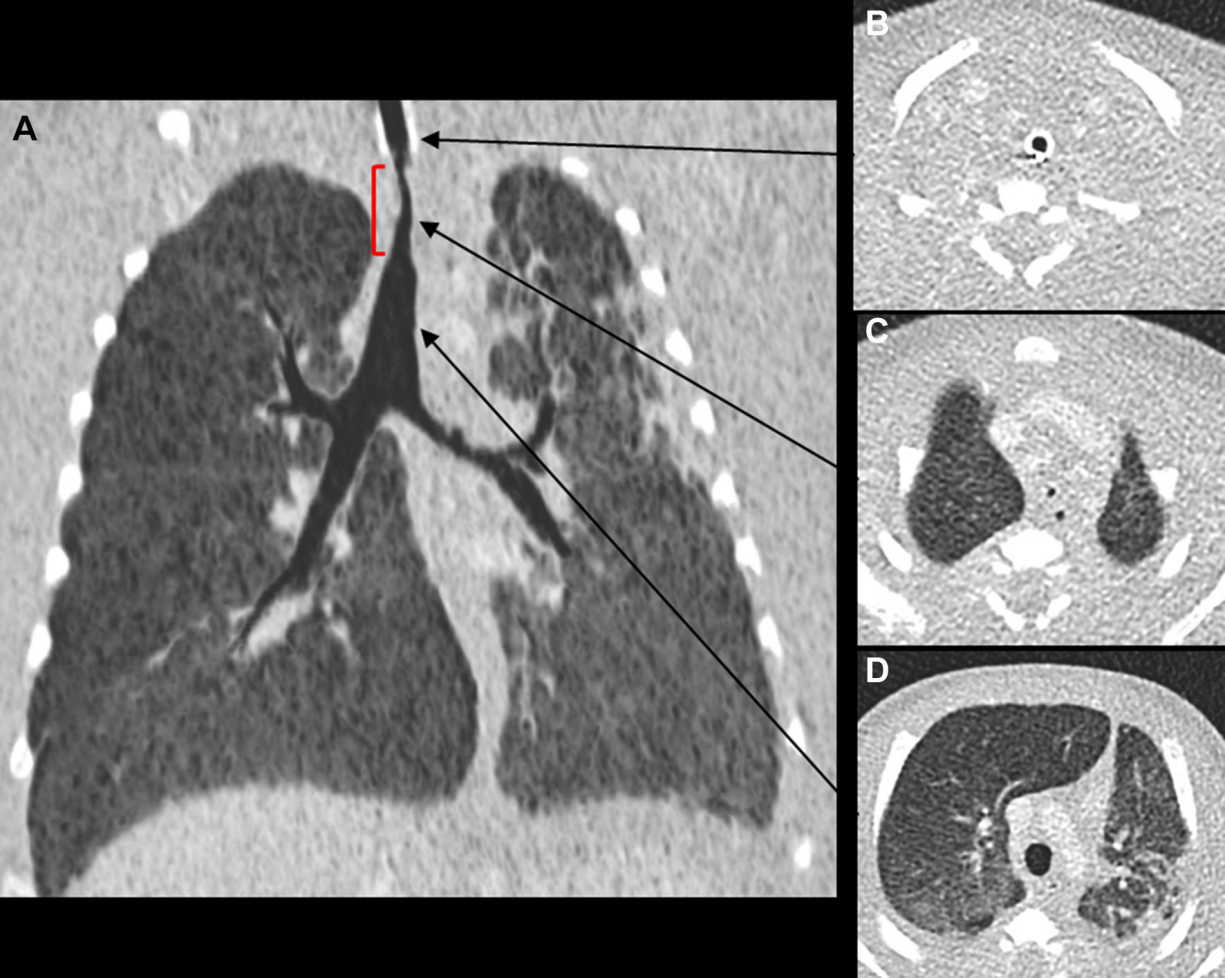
Antemortem CT thorax obtained 1 month prior to death. (A) A coronal 5 mm minimum intensity projection (miniP) image, viewed on lung windows, demonstrates the tight tracheal stenosis immediately distal to the endotracheal tube (red square bracket). Axial CT sections obtained (B) above, (C) at the level of the stenosis and (D) inferior to the stenosis show the relative narrowing of the trachea in relation to the intubated and non-stenosed sections (arrows).
